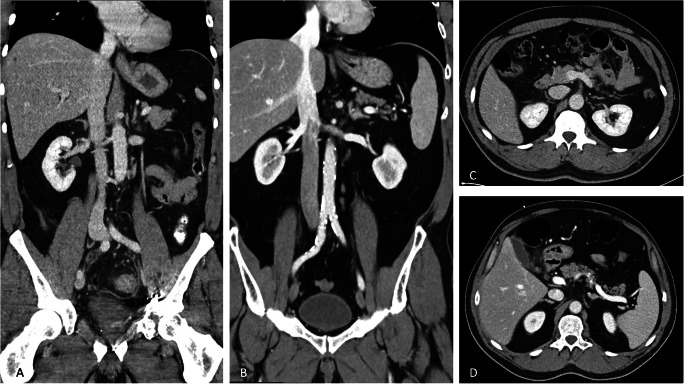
Example of axial and coronal reconstruction of abdominal conventional CT (A and C) and PCCT (B and D) for the depiction of subjective image impression. DLP was 413.38 and 453.98 mGy*cm for PCCT and conventional CT, respectively. Both examinations were rated “very good” by all examiners (general image quality PCCT: 4.75 ± 0.5; general image quality conventional CT: 4.75 ± 0.5)
